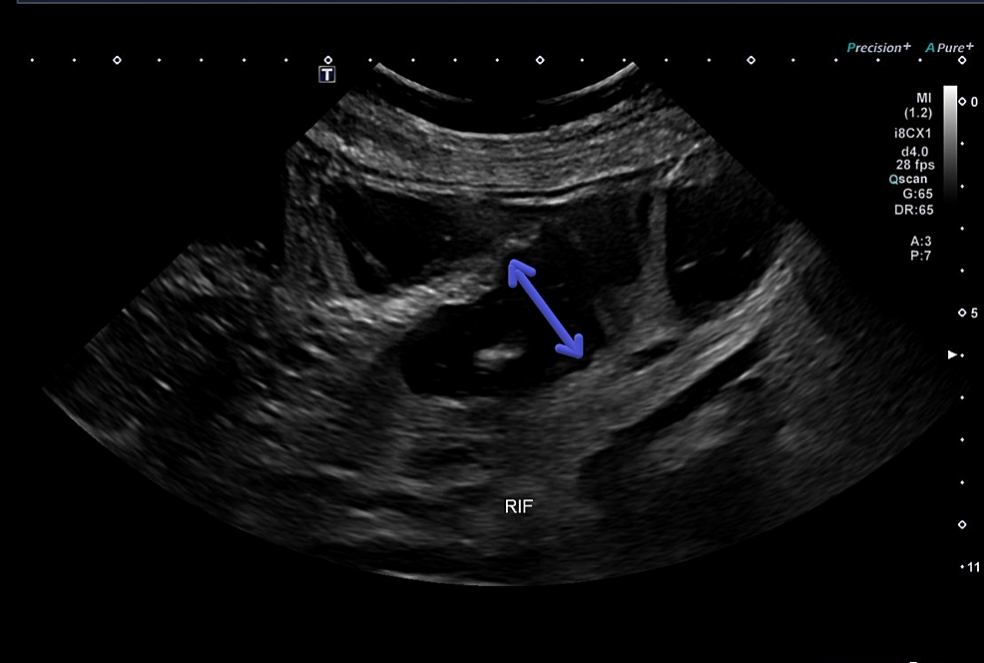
Ultrasound view image demonstrate prominent bowel loops are noted (double-headed arrow), with to and fro movement detected during the ultrasound examination
